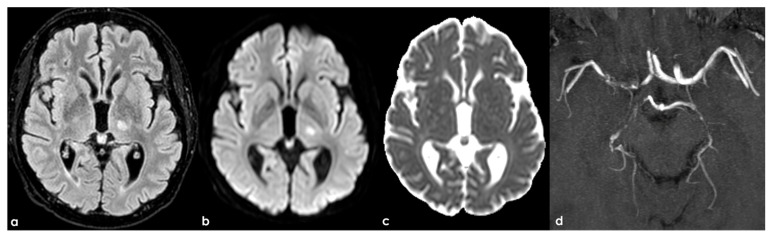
Brain MRI and MRA of the same patient as shown in Figure 4 (panel (a), axial FLAIR; panel (b), axial DWI; panel (c), axial ADC; panel (d), MRA in axial plane with MIP/MPR protocol).
